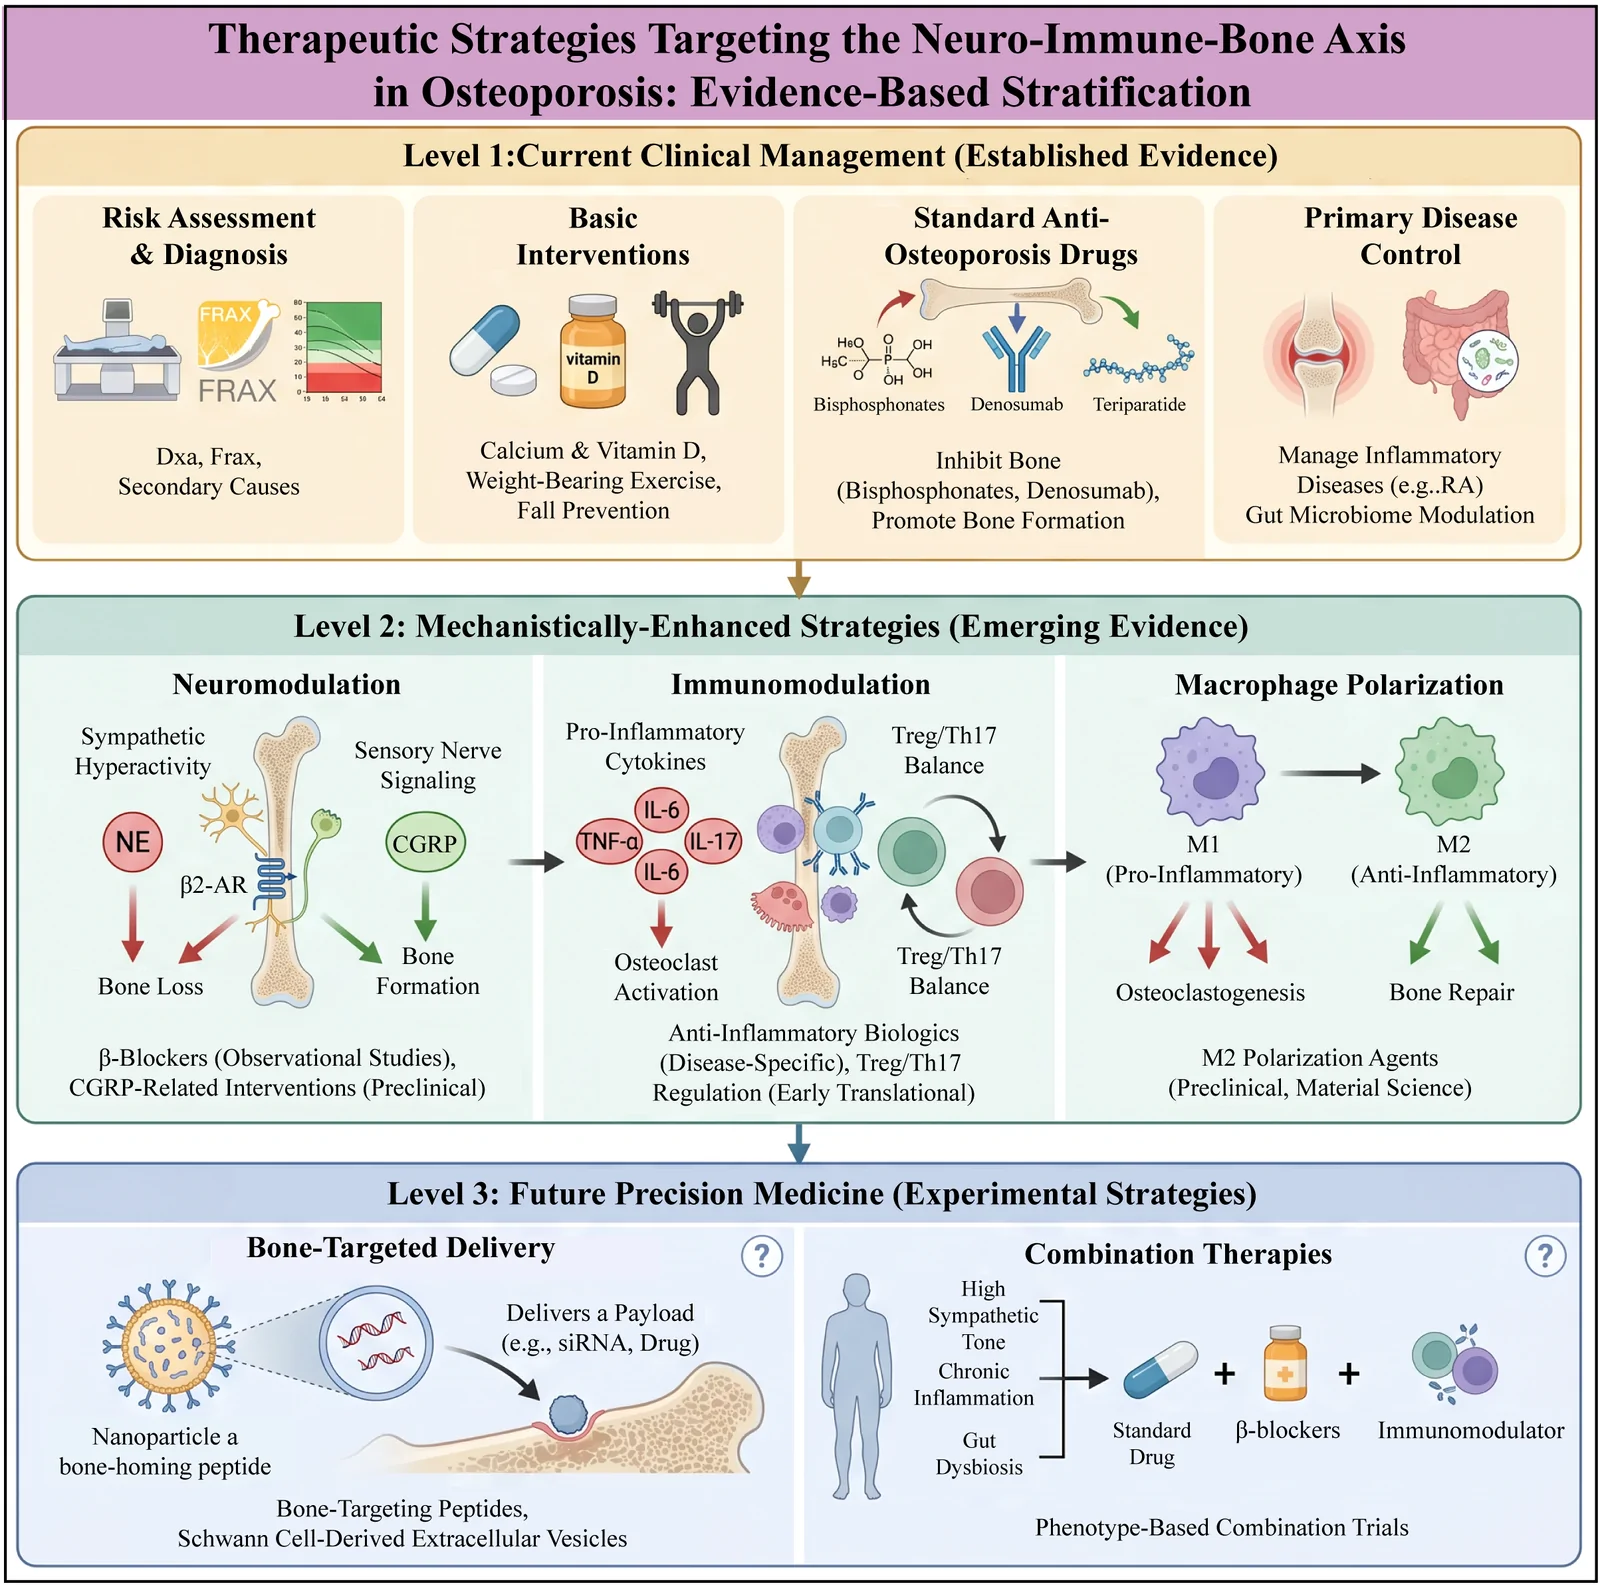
Evidence stratification framework for interventions targeting the neuro-immune-bone axis in osteoporosis. Level 1: Current clinical management. This category encompasses DXA/FRAX assessment, calcium and vitamin D management, exercise, fall prevention, primary disease control, and guideline-recommended medications, all supported by robust clinical evidence. Level 2, Disease-specific or exploratory interventions: includes anti-inflammatory biologics, sympathetic nervous system modulation, CGRP-related interventions, Treg/Th17 regulation, and macrophage remodeling. Except for anti-inflammatory biologics used for specific inflammatory diseases, most are in observational, preclinical, or early translational stages. Level 3, Experimental precision interventions: includes bone-targeted delivery, extracellular vesicles, bone-homing peptides, and phenotype-oriented combination therapies, currently mainly used for mechanistic and technical research, requiring further validation of efficacy and safety. Abbreviations: CGRP, calcitonin gene-related peptide; DXA, dual-energy X-ray absorptiometry; FRAX, Fracture Risk Assessment Tool; Th17, T helper 17 cells; Treg, regulatory T cells.
